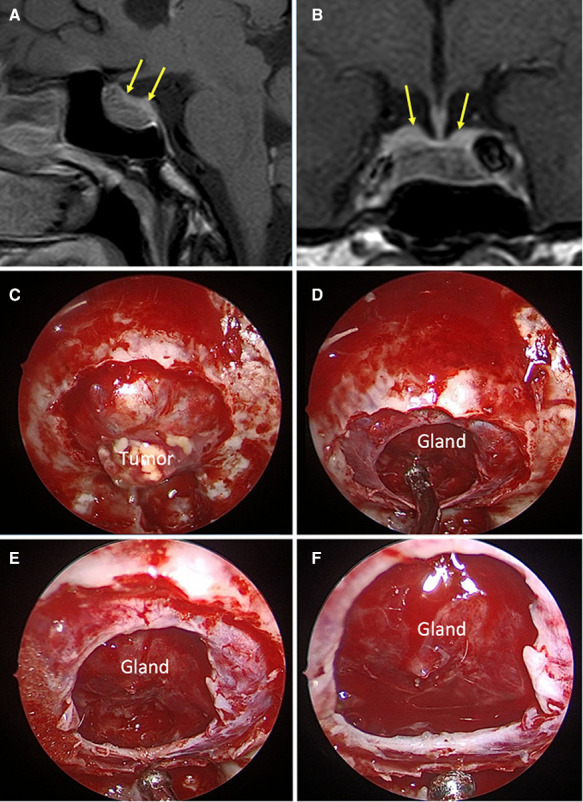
Strong sellar barrier.* A 42-year-old male patient, with GH produce macroadenoma. (A,B) Preoperative MRI: the yellow arrows indicate the barrier that captures contrast with a thickness greater than 1 mm. (C–F) Intraoperative images: the barrier constituted by the gland can be seen.
